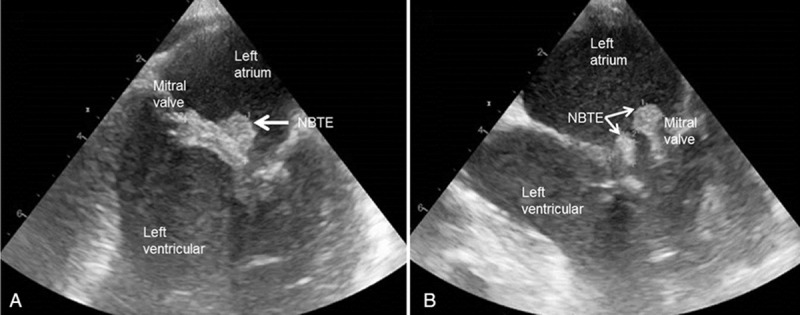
Transesophageal echocardiography, long-axis views, showing nonbacterial thrombotic endocarditis in a patient with pancreatic cancer. NBTE = nonbacterial thrombotic endocarditis.
